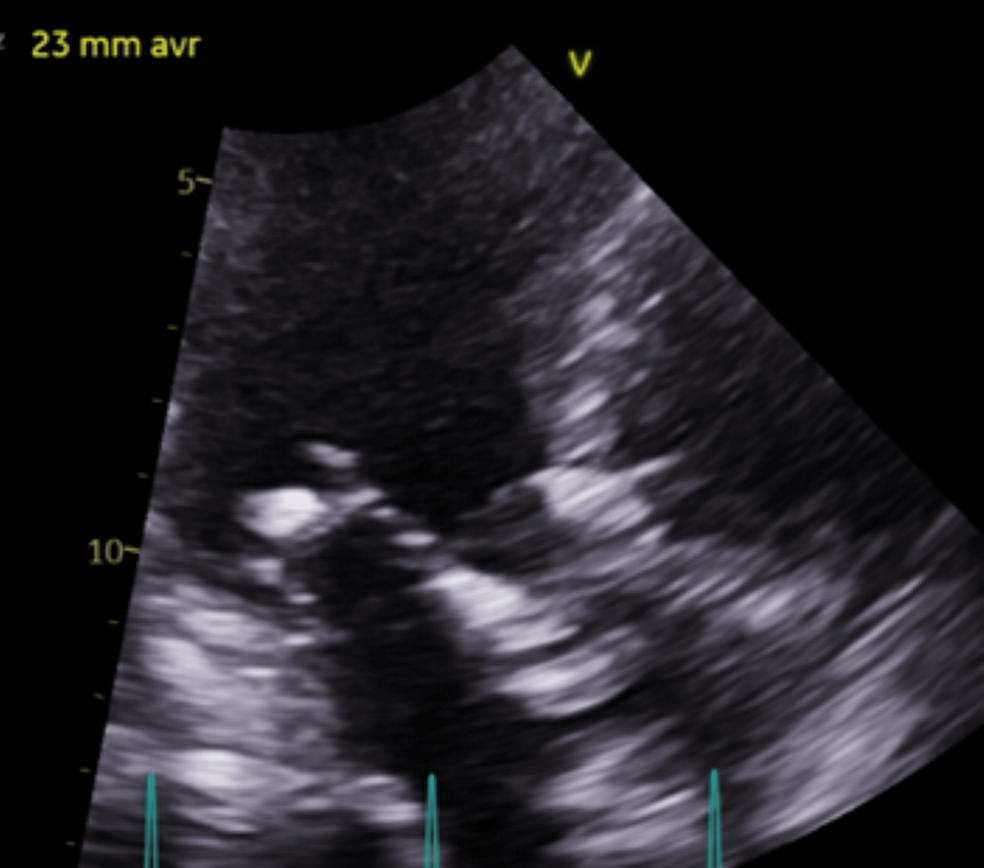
Six Weeks Post TAVR. Thrombosed Valve while on Apixaban Ultrasound three-chamber long-axis view showing thickening of bioprosthetic valve leaflets. TAVR: transcatheter aortic valve replacement
